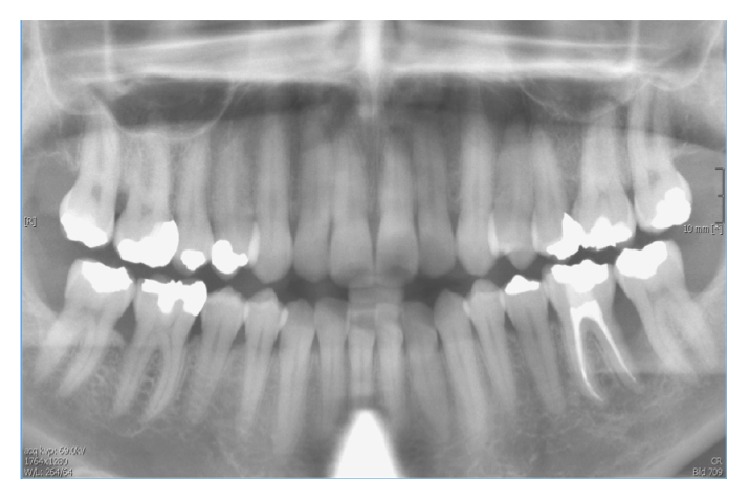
Panoramic radiograph. The periodontal defects, especially at tooth 36, showed progressive periodontal healing 24 months after regenerative periodontal treatment.
